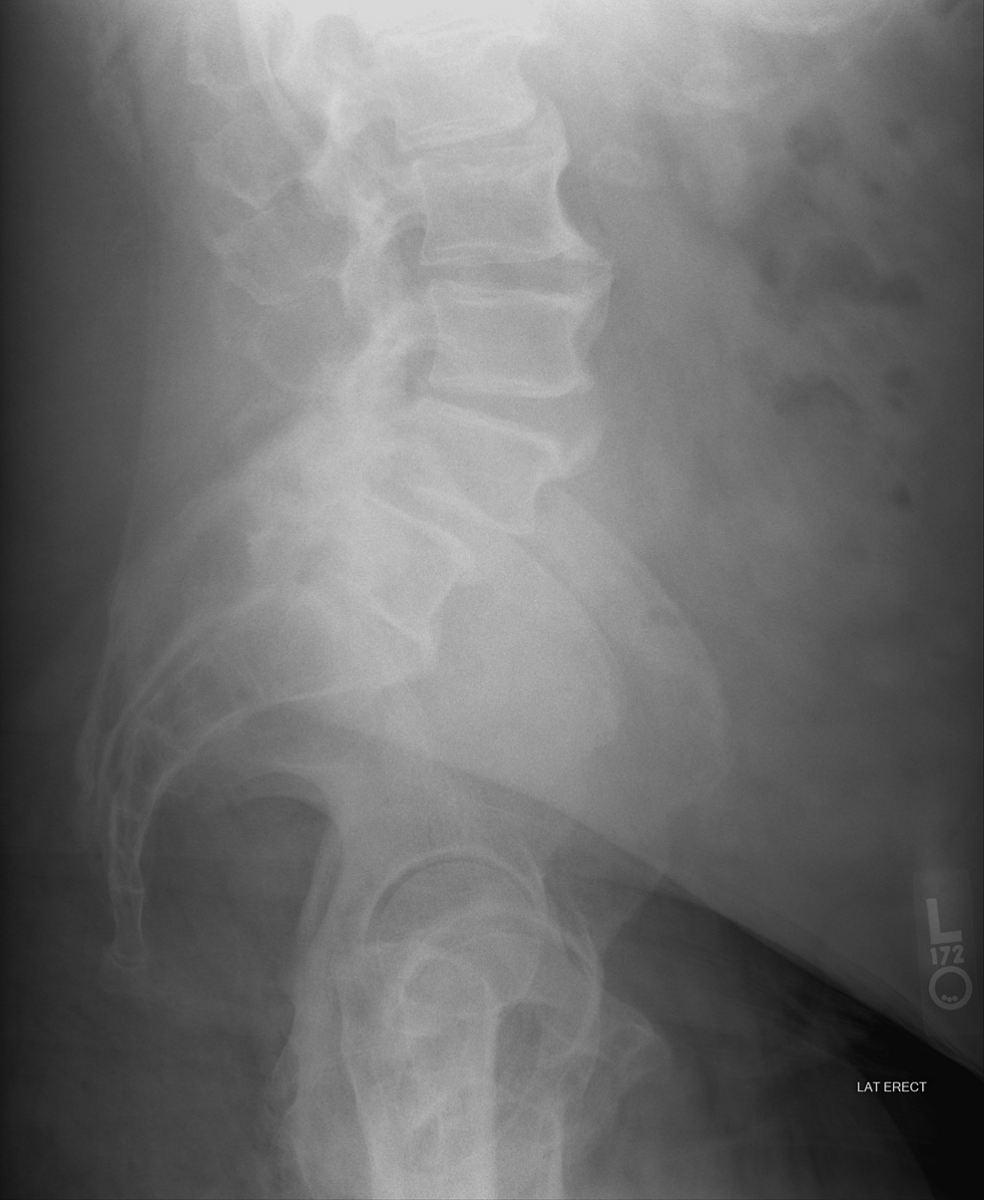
Preoperative lateral lumbar spine X-ray demonstrated an unstable L4-5 spondylolisthesis with significant facet hypertrophy at L4-L5.
